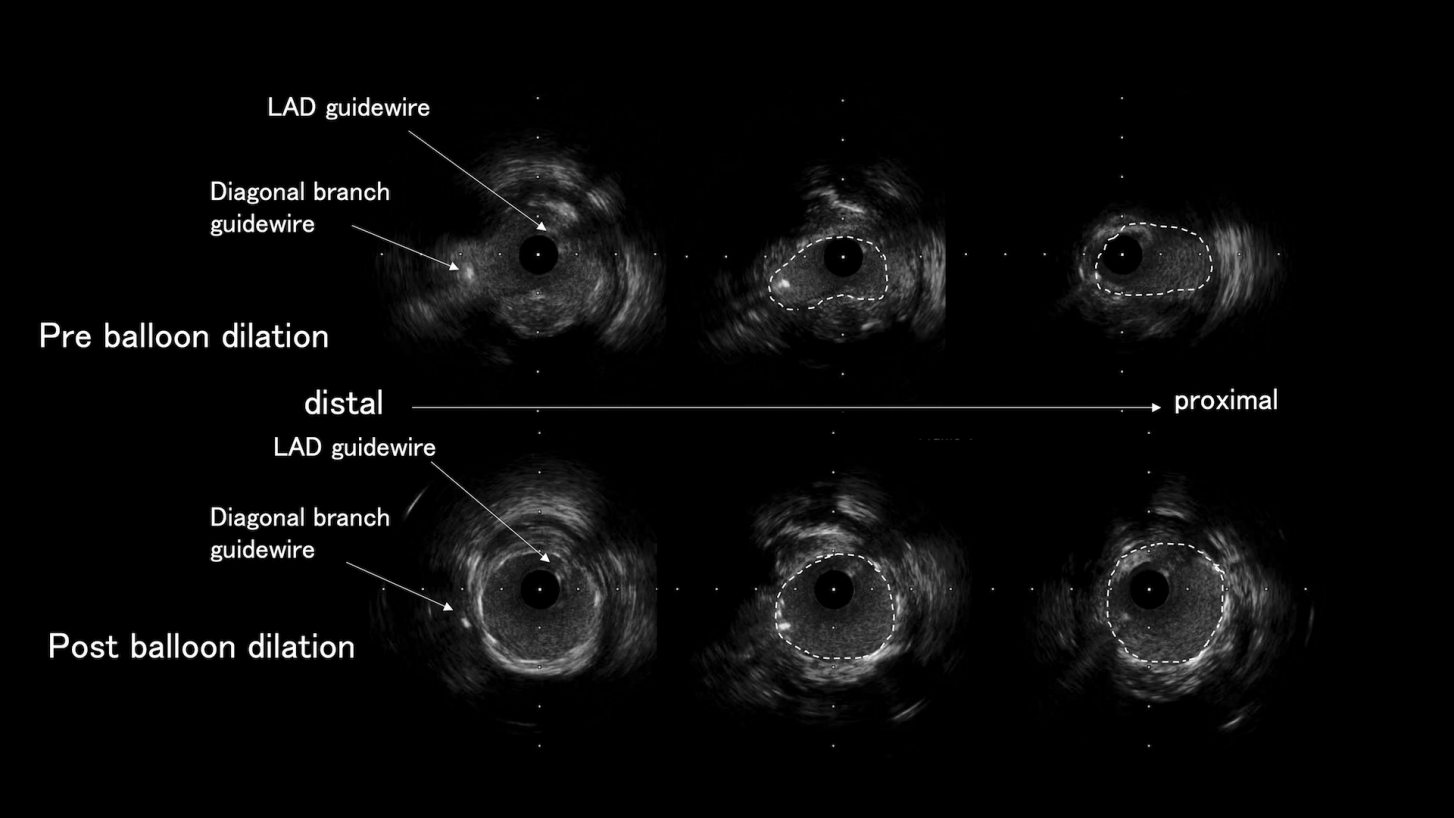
Imaging of intravascular ultrasound at pre balloon dilation (upper side) revealed that two guidewires in stenosis were very close to each other. Imaging of intravascular ultrasound at post balloon dilation (bottom side) indicated that the release of stenosis reduced the wire bias.
